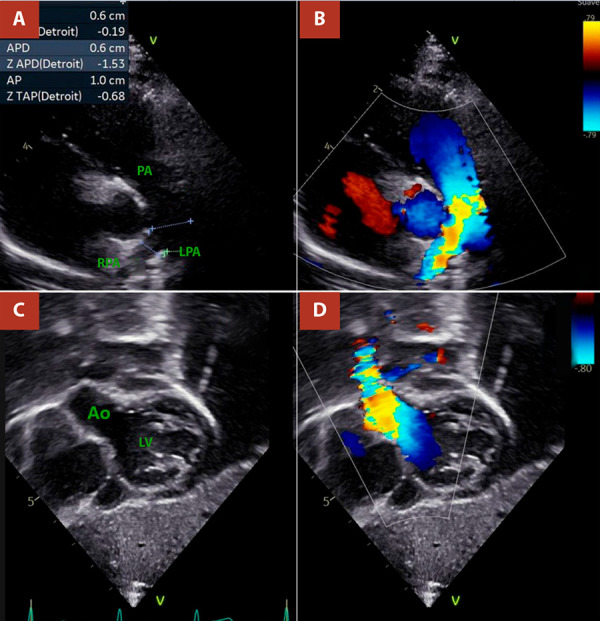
Follow-up echocardiography of Case 02 at 16 months. A) and B) Short-axis views showing no flow acceleration at the level of the pulmonary branches; right pulmonary artery diameter (z-score −1.5). C) and D) Subcostal view of the ascending aorta showing a reported maximum gradient <9 mmHg.
